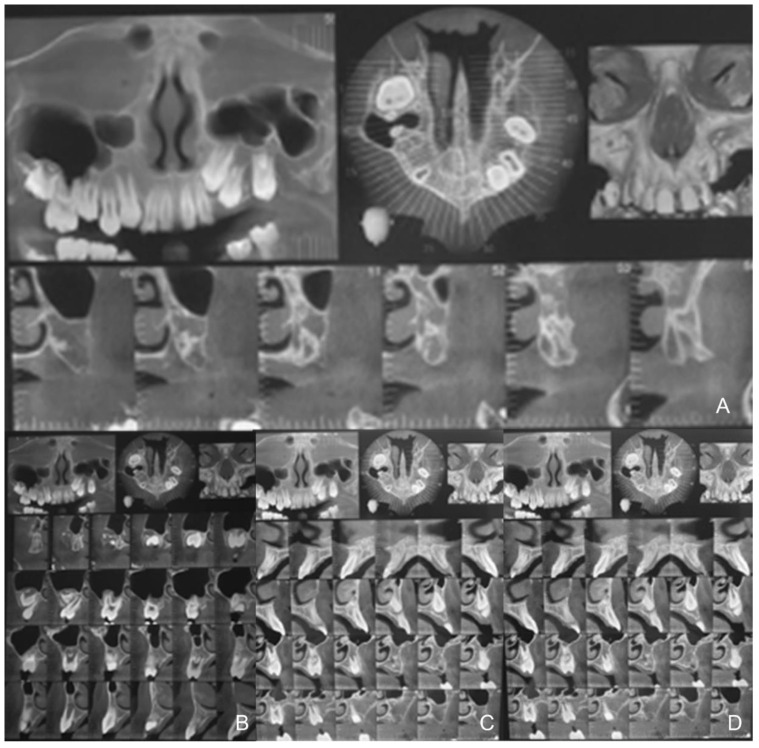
CBCT evaluation of the degree of ossification and miniscrew positioning.
